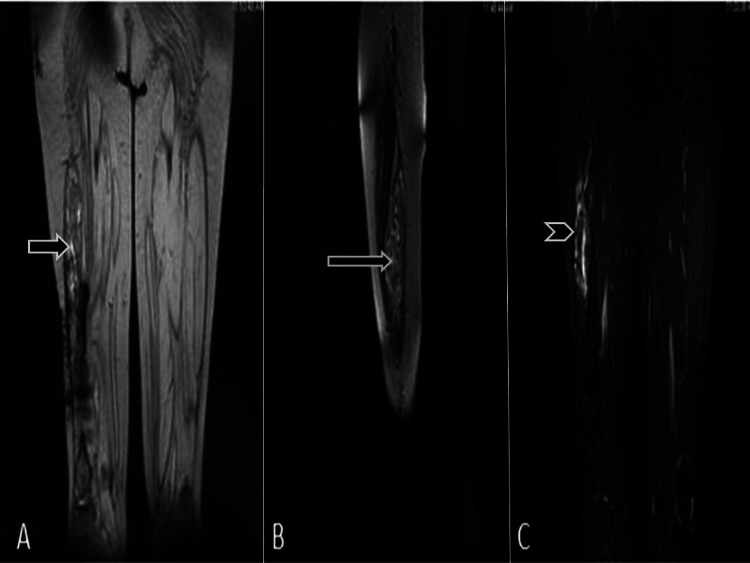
MRI of the patient Images showing coronal T2 (A) T2 sagittal (B) and STIR sequences (C) that predominantly show hyperintense lesions with a fatty component in the right thigh, which is suppressed on STIR. Proximal femur fracture with artifacts from plate and screw fixation is also seen. Diffuse atrophy of all muscles of the proximal thigh is noted MRI: magnetic resonance imaging; STIR: short-tau inversion recovery
